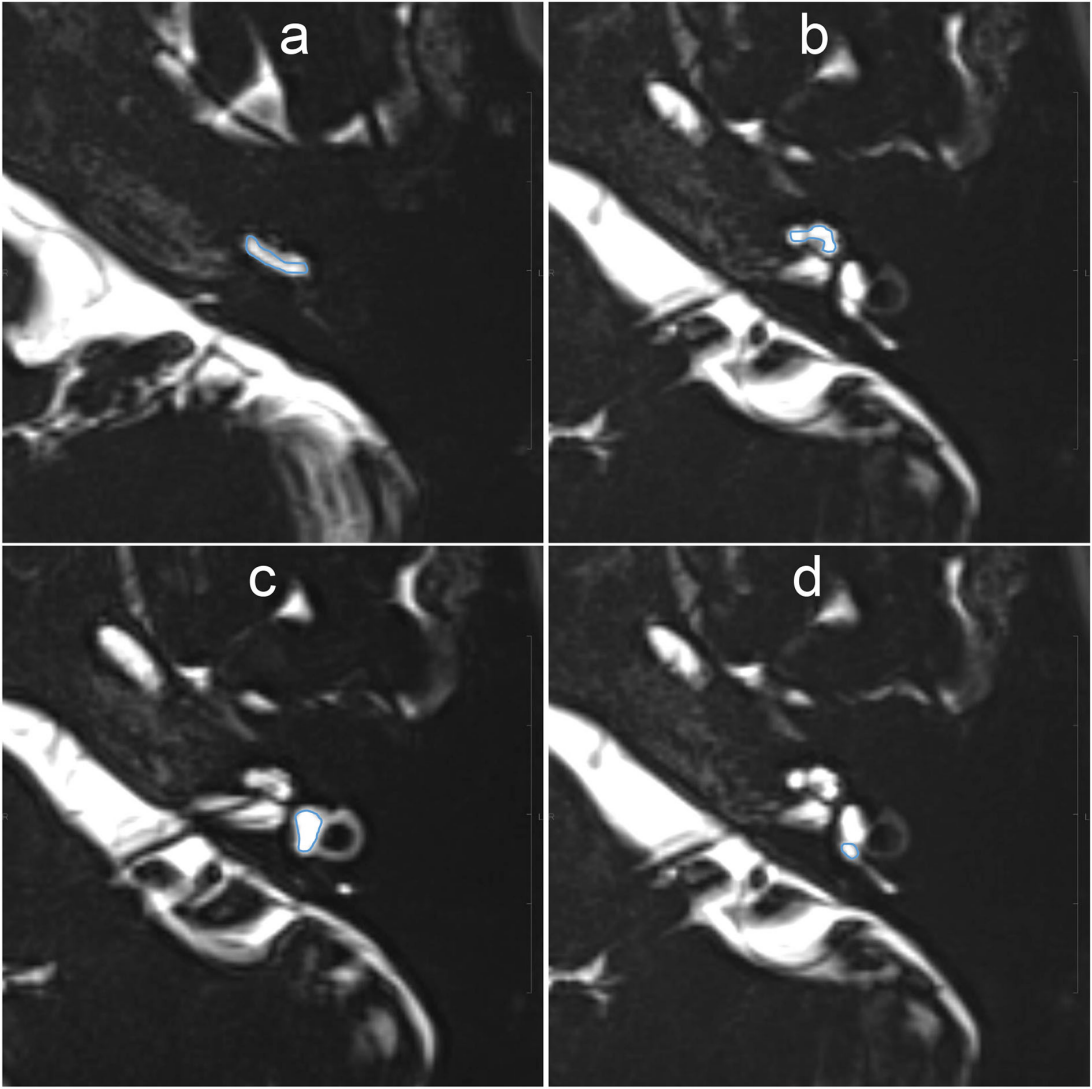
Example images for setting of the region of interest (ROI) on MR images with an echo time (TE) of 540 ms. The scala tympani of the basal turn of the cochlea (a), whole apical–middle turn (b), vestibule (c), and the ampulla of the posterior semicircular canal (d) appeared contoured. The ROIs were then copied onto the other TE images. Each signal–intensity ratio was calculated using the signal value of the contralateral inner ear as a control.
